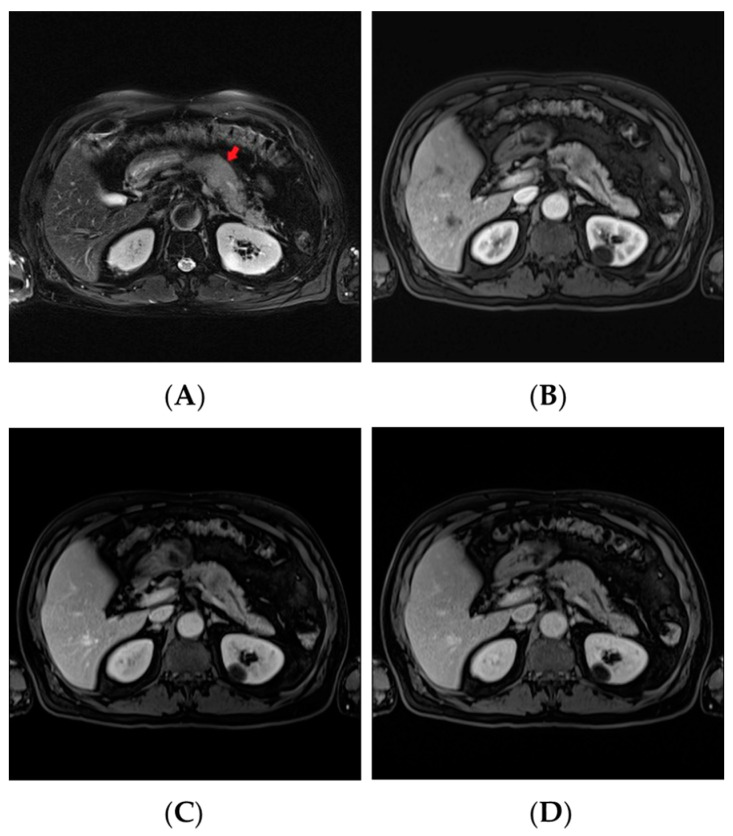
(A) Pancreatic magnetic resonance image (MRI) showed a pancreatic body hypovascular lesion (red arrow), approximately 3.3 cm, with peripancreatic invasion. (B) Arterial phase. (C) Portal venous phase. (D) Delayed phase.
